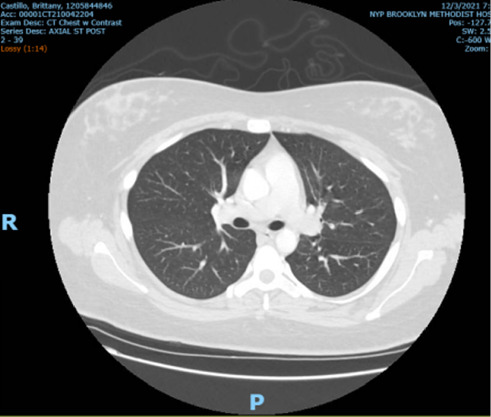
CT chest with contrast which did not demonstrate and remarkable findings.
